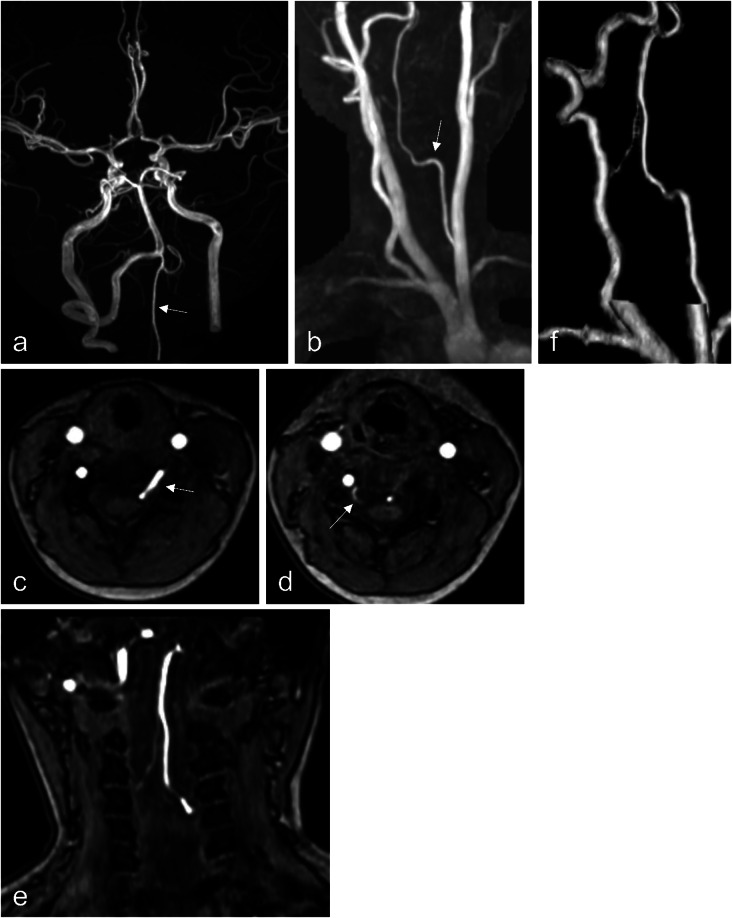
Head and neck MRA images using a 3 T magnetic resonance imaging scanner. a: Anteroposterior projection of a maximum intensity projection (MIP) image showing the absence of a normal left VA course with visualization of the developed anterior spinal artery as a collateral pathway (arrow). b: Lateral MIP projection showing the ectopic course of the left vertebral artery (arrow). c: MRA source image at the level of the C4–C5 vertebrae shows the entry of the left VA into the spinal canal via the C4–C5 intervertebral foramen (arrow). d: MRA source image at the level of the C3–C4 vertebrae shows the entry of the small branch (radiculomedullary artery) originating from the right vertebral artery into the spinal canal via the C3–C4 intervertebral foramen (arrow). e: Coronal section of the MR angiographic source image. Two blood vessels ascending within the spinal canal converge at the C1 level to form an anterior spinal artery. f: A volume-rendered image focusing on the vertebral artery is shown, with the above vascular anatomy clearly defined
